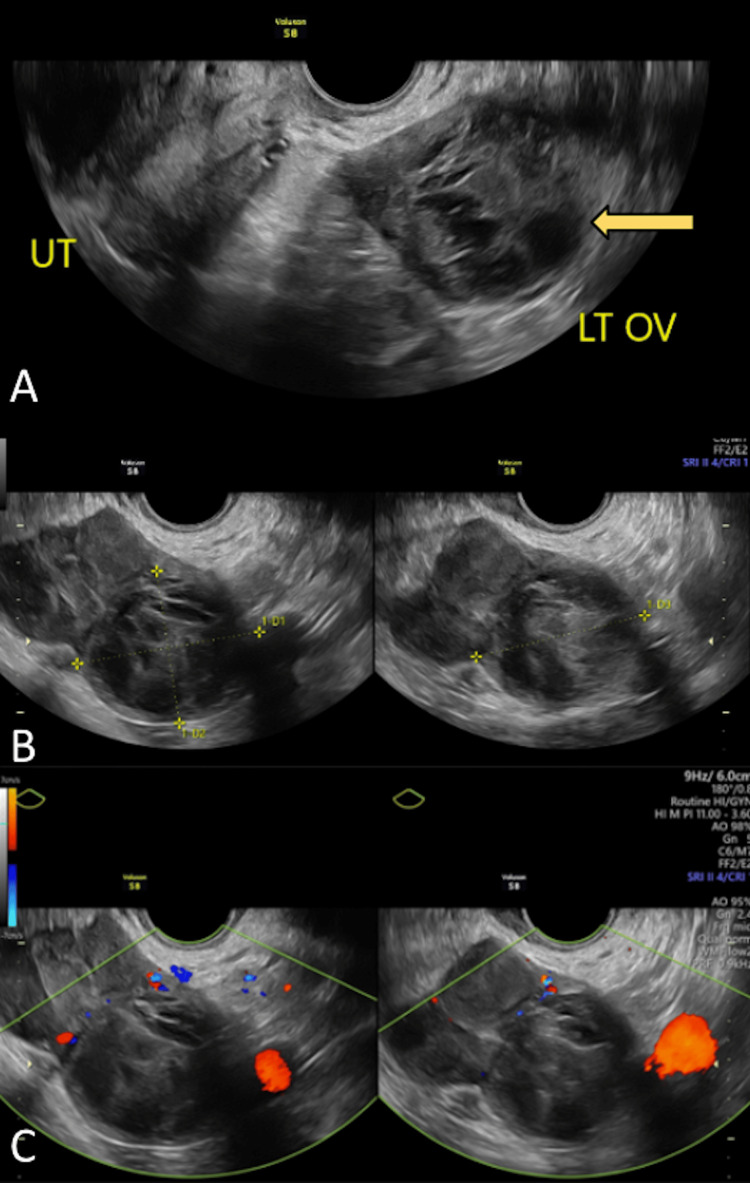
(A-C) Transvaginal Doppler duplex ultrasound of left-sided ovarian mass Figure 1A depicts a gray-scale image of a heterogeneous left ovarian mass with lobulated borders (yellow arrow). Figure 1B demonstrates a gray-scale image of a left ovarian mass measured at 4.4 x 6.7 x 4.5 centimeters (yellow dotted lines). Figure 1C shows a spectral and color Doppler image of the left ovarian mass demonstrating internal blood flow (green outline). LT OV, left ovary; UT, uterus
